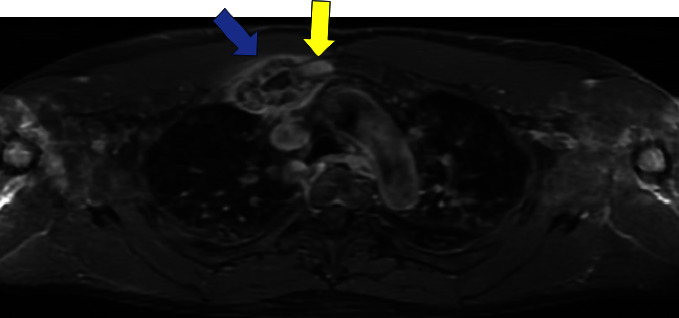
Axial T1-weighted fat-saturated postcontrast MRI of the upper thorax showing enlargement of the right first rib (blue arrow) at the costochondral junction involving the costomanubrial articulation. The chondral rib is enlarged with a thick osseous rim that intrinsically enhances. The adjacent marrow of the manubrium and surrounding soft tissues enhances (yellow arrow).
